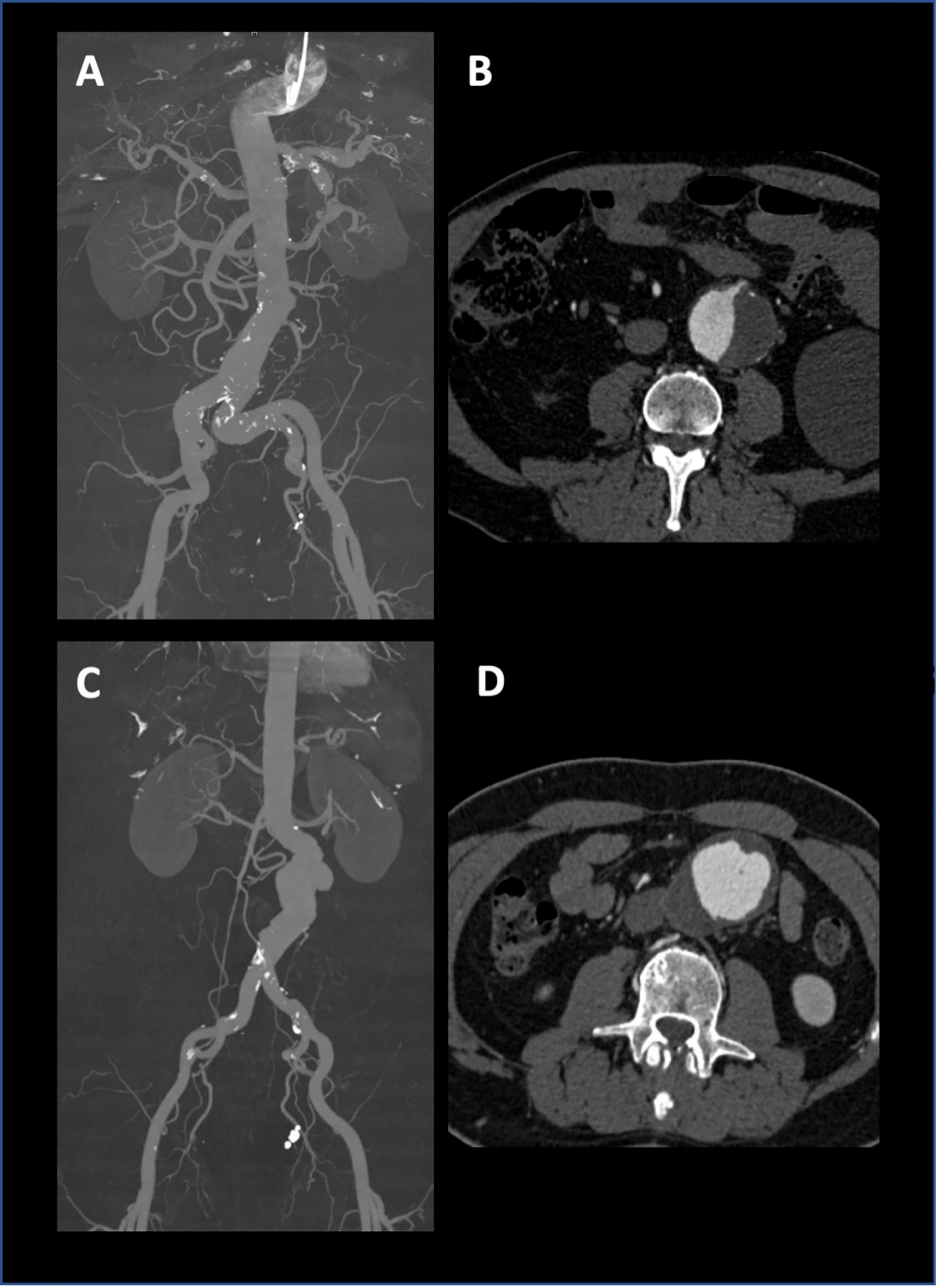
Representative CTA images of a catheter-directed CTA (coronal maximum intensity projection in A, transversal image after i.a. contrast application in B), and in comparison of an intravenous CTA (coronal maximum intensity projection in C, transversal image after i.v. contrast application in D).
